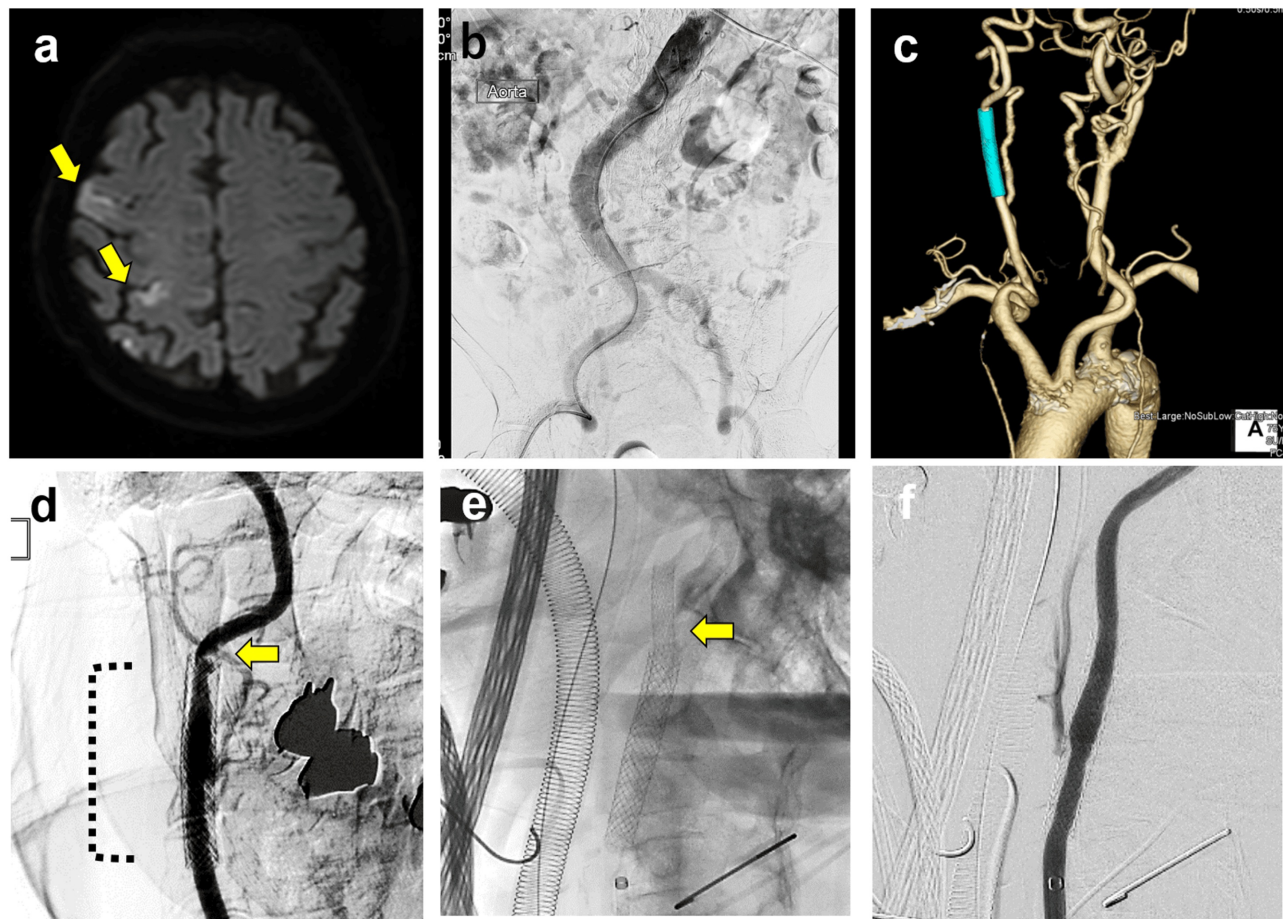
Symptomatic severe re-stenosis of the right internal carotid artery after the previous carotid artery stenting (case two) a) Head diffusion-weighted magnetic resonance imaging (MRI-DWI) demonstrates an acute cerebral infarction in the right frontal and parietal subcortical white matter (arrows). b) Digital subtraction angiography (DSA) reveals severe vascular tortuosity from the abdomen to both lower extremities. c) Cervicothoracic 3D-CT angiography demonstrating marked calcification of the thoracic aorta and significant tortuosity of the cervical vessels. d) DSA showing severe stenosis of the right internal carotid artery (ICA) (arrow), distal to the previously placed carotid stent (dotted line). e) Overlapping stent fully covering the stenotic lesion of the right ICA (arrow). f) DSA of the right common carotid artery confirming successful revascularization following carotid artery stenting.
